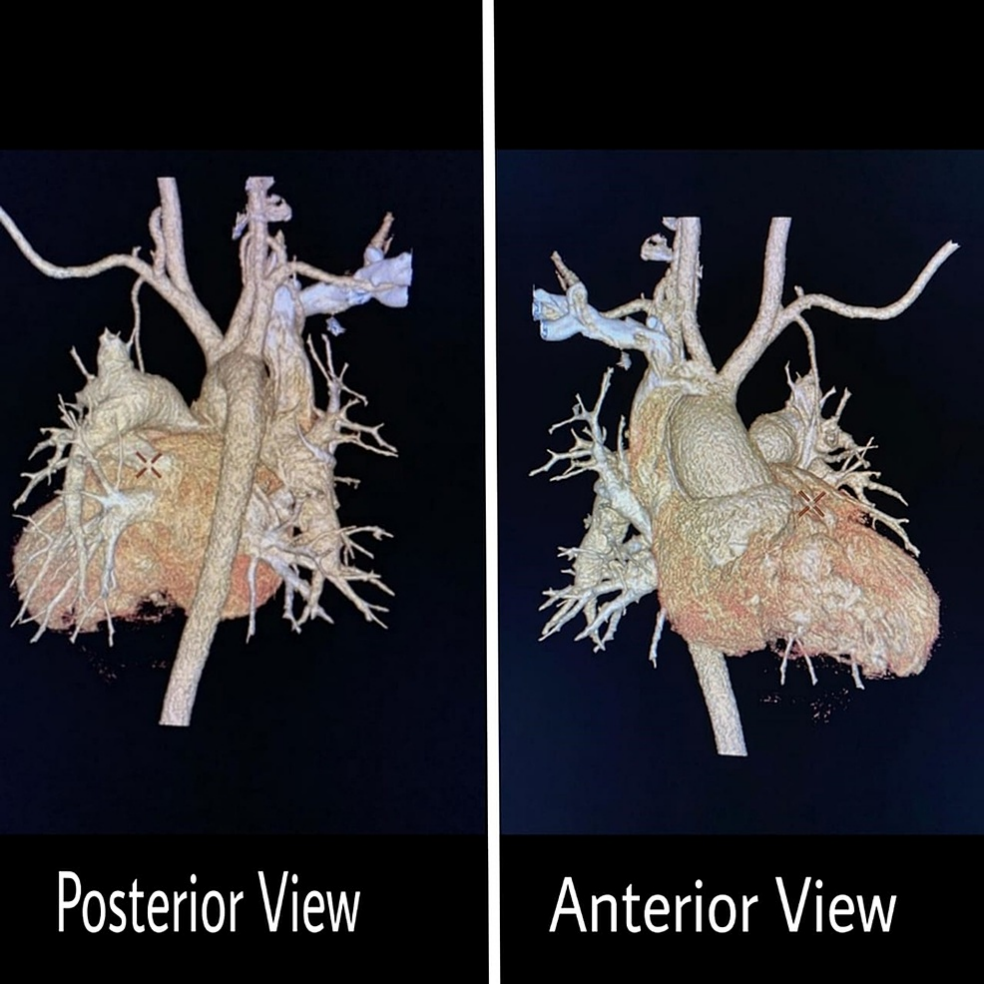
Volume rendering CT cardiac dynamic study showing a posterior and anterior view of the heart. Image credits: Dr. Viral B. Patel.
